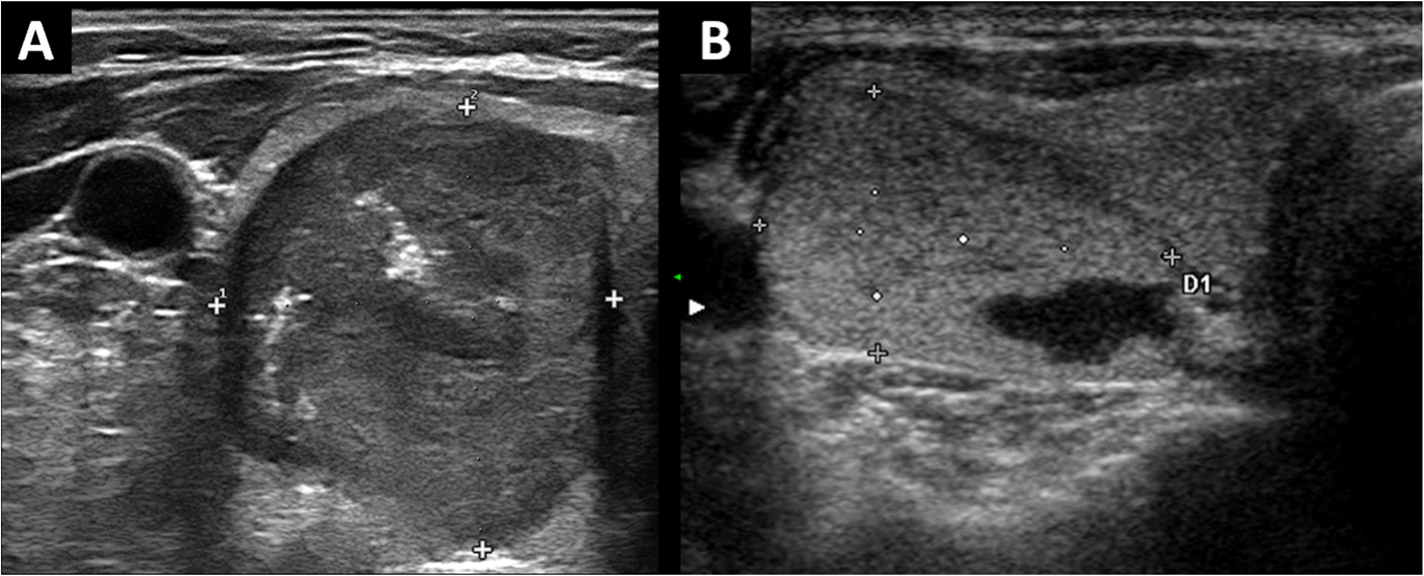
Different ultrasound presentations of MTC. (A) hypoechoic nodule with calcifications, classified at ultrasonography as suspicious. (B) mixed-spongiform nodule with hypoechoic halo, non-suspicious at ultrasonography.
